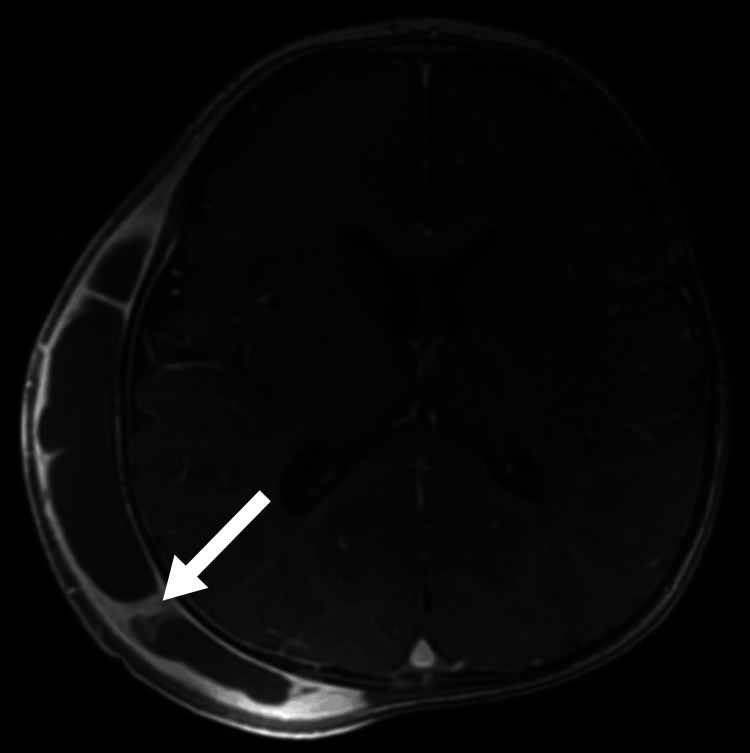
Post contrast axial T1 WI MRI of the brain revealed subgaleal fluid collection with markedly enhancing wall and internal septations
